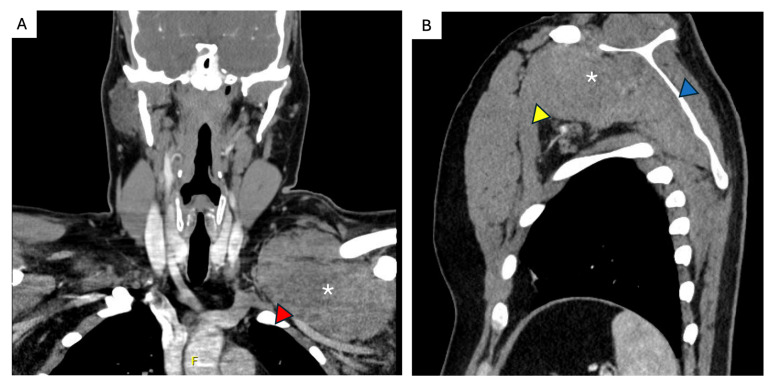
Shoulder girdle desmoid tumor. (A) A 27-year-old male patient with a history of left glenohumeral traumatic dislocation 3 years prior was referred for a 12-month history of a sporadic DT of the left shoulder girdle (asterisk), stable in size but with worsening pain and restricted range of motion. Coronal CT shows compression of the axillary vessels (red arrow). (B) Sagittal view shows an intimate relationship with neurovascular structures (yellow arrow) and loss of fat plane with the subscapularis muscle (blue arrow). He underwent first-line medical therapy, with symptomatic improvement.
